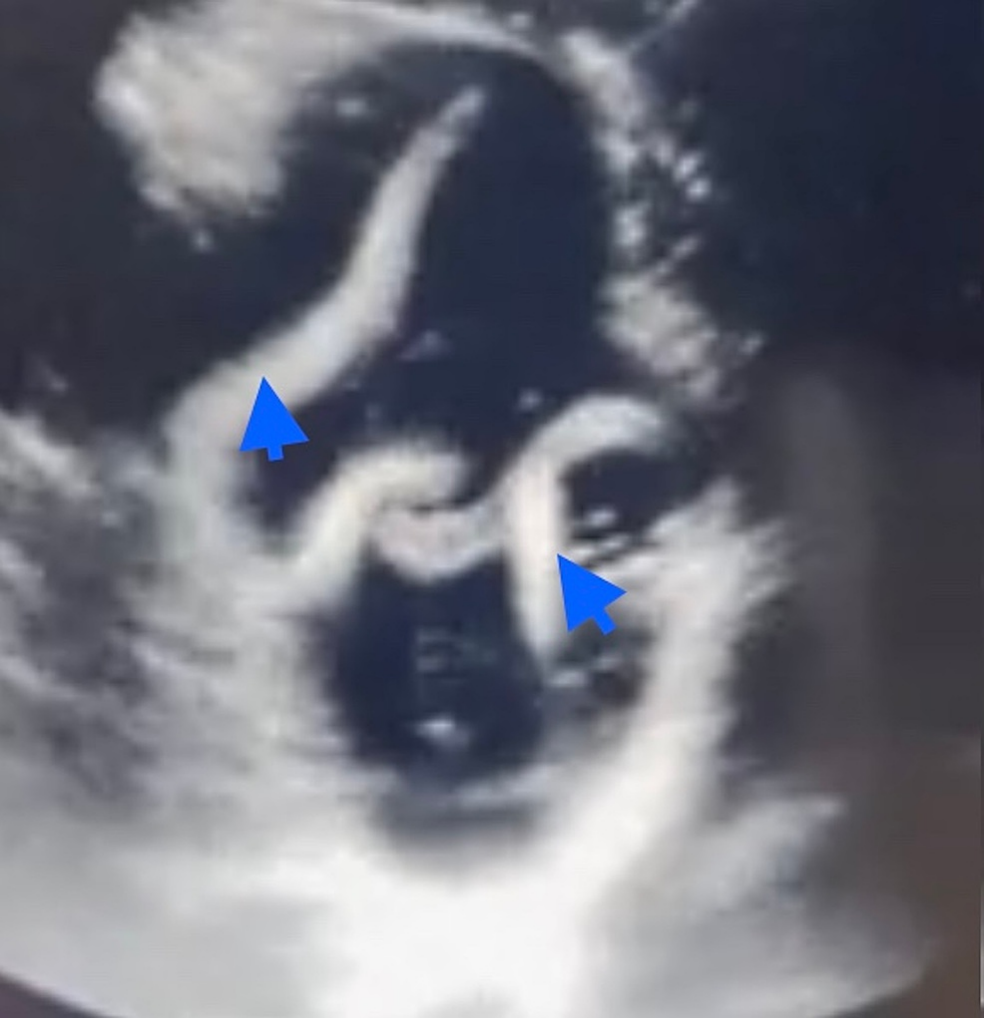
Abdominal ultrasound showing roundworms (blue arrows) in the jejunum
